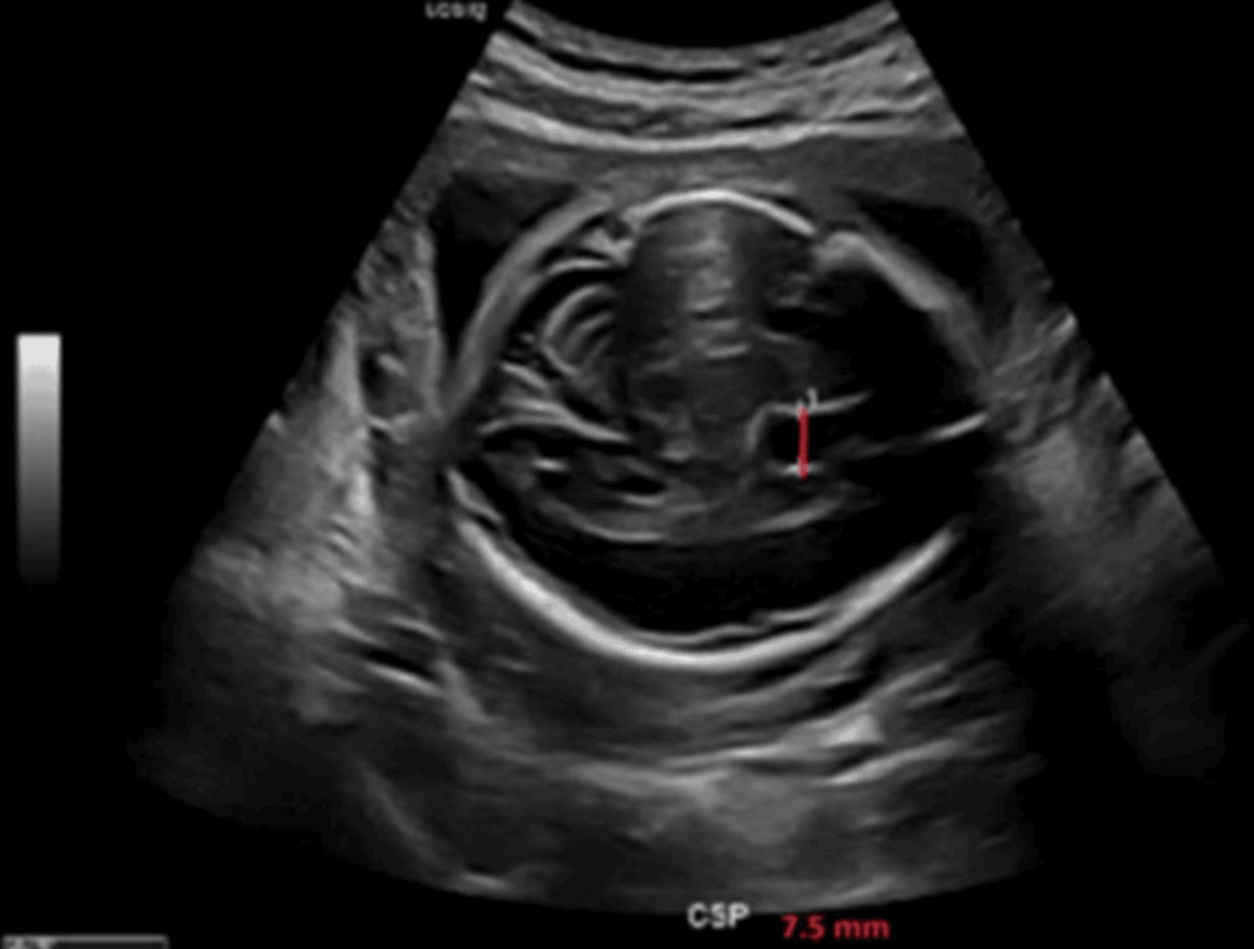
Ultrasound view of the fetal brain at 24 weeks and 4 days of gestation demonstrating the CSP (red line) measuring 7.5 mm, corresponding to the 95th percentile for gestational age CSP: cavum septum pellucidum
